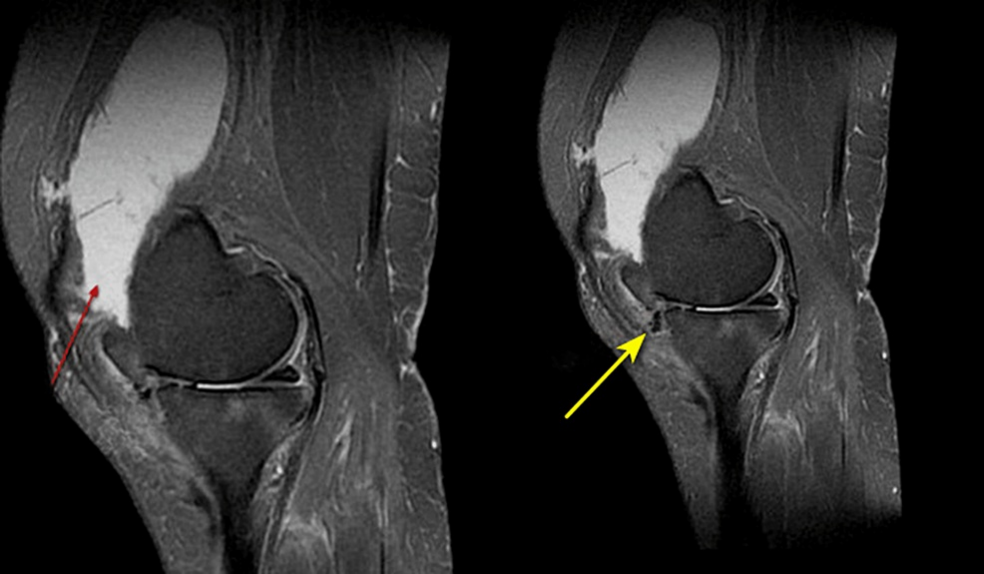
Sagittal PDFatSat MRI showing suprapatellar joint effusion (red arrow) and tear of the anterior horn of the medial meniscus (yellow arrow). PDFatSat: proton density fat saturation, MRI: magnetic resonance imaging
